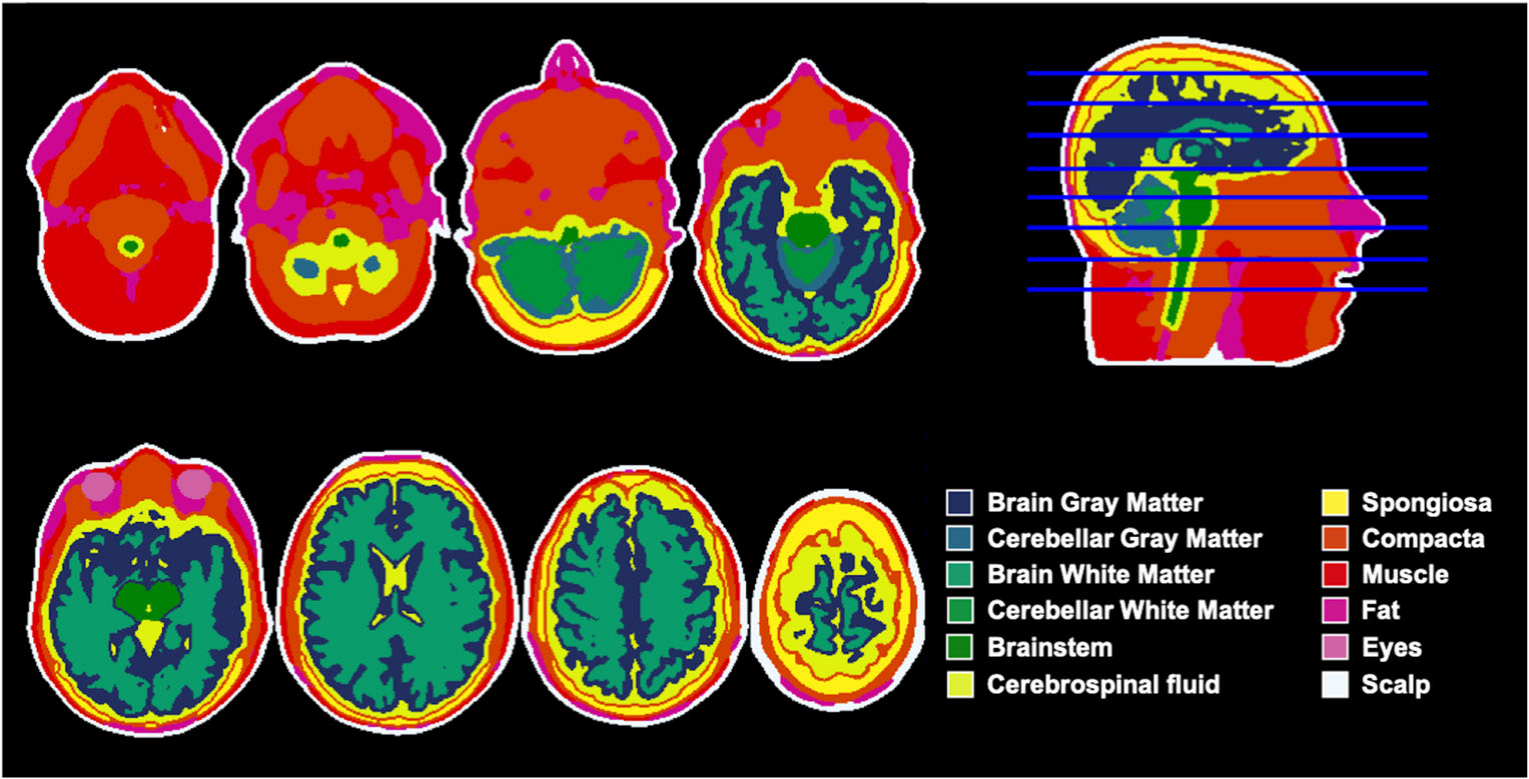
Sample output of whole-head automated segmentation produced by MR-TIM. The results obtained on the MR image from subject S01 are shown in volumetric space. The 12 tissue classes are represented with different colours
